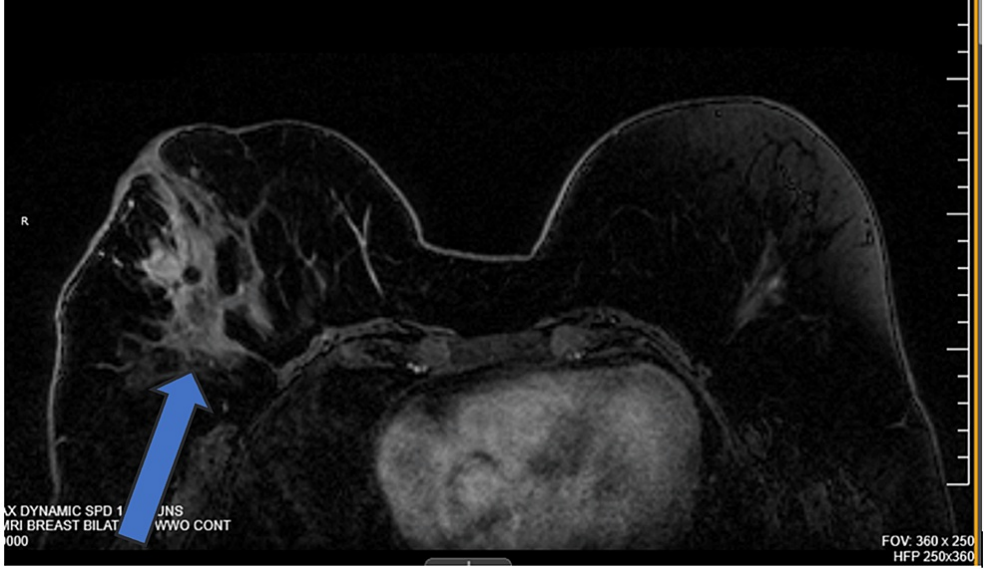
MRI of the bilateral breast with and without contrast showing an irregular mass on the right breast.
